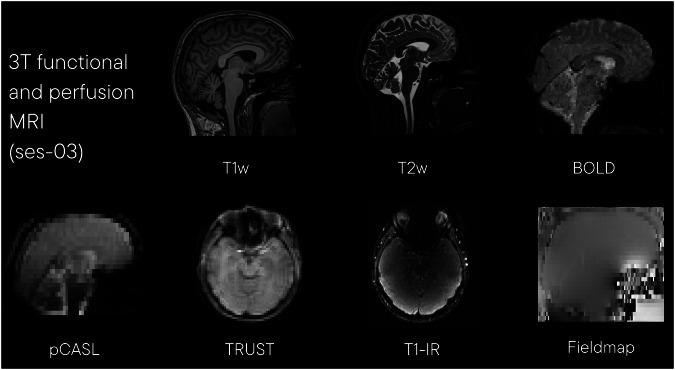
Example images from session 03, 3 T functional and perfusion MRI. T1w: T1-weighted structural image (acquired with MPRAGE); T2w: T2-weighted turbo spin echo; BOLD: blood oxygen level dependent functional MRI; pCASL: pseudo-continuous arterial spin labelling; TRUST: T2 relaxation under spin tagging; T1-IR: T1 inversion recovery.
